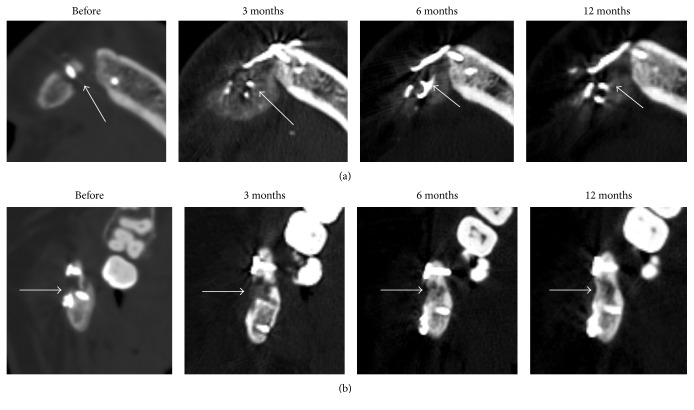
CT scans of the patient's mandible in the frontal region (a) and within the ramus on the right side (b) prior to the operation and 3, 6, and 12 months after surgery. Arrows indicate nonunions (before operation) and the sites of gene-activated bone substitute implantation.
